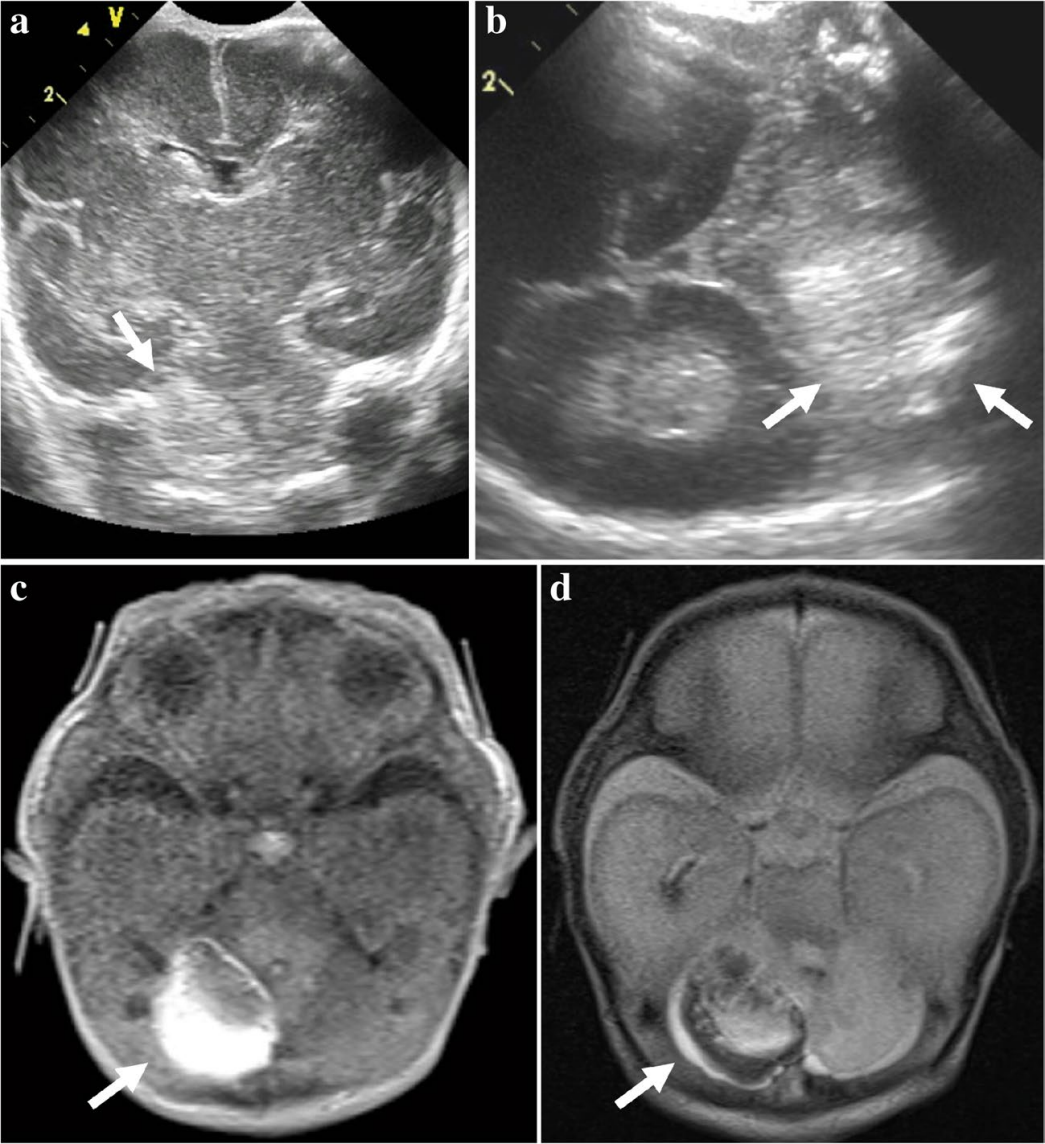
Representative case of type 5 cerebellar hemorrhage in a preterm girl (26 + 3 weeks, 1,020 g). a, b Oblique coronal anterior fontanelle (a) and axial left mastoid (b) views show large, mass-like echogenic lesion (*arrows*) in the right cerebellar hemisphere. c Axial T1-weighted magnetic resonance image acquired 10 days after the ultrasonography shows mixed high and iso-to-low signal intensity hemorrhage in the right cerebellar hemisphere. d Axial T2-weighted magnetic resonance image shows a complex lesion with mixed signal intensities in the right cerebellar hemisphere
